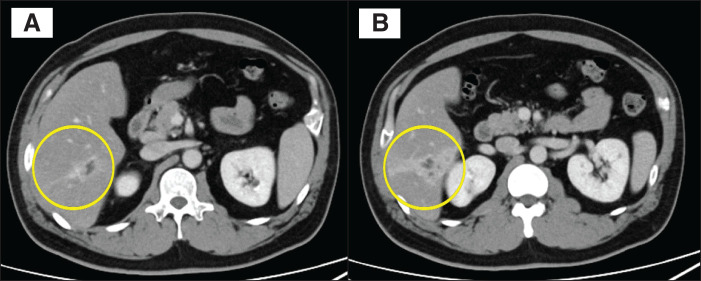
Computed tomography image (portal phase). A low-density area with contrast enhancement in the periportal wall and a filling defect are visible along the ventral region of segment 6; (A) is an axial slice on the cranial side and (B) is an axial slice on the caudal side.
